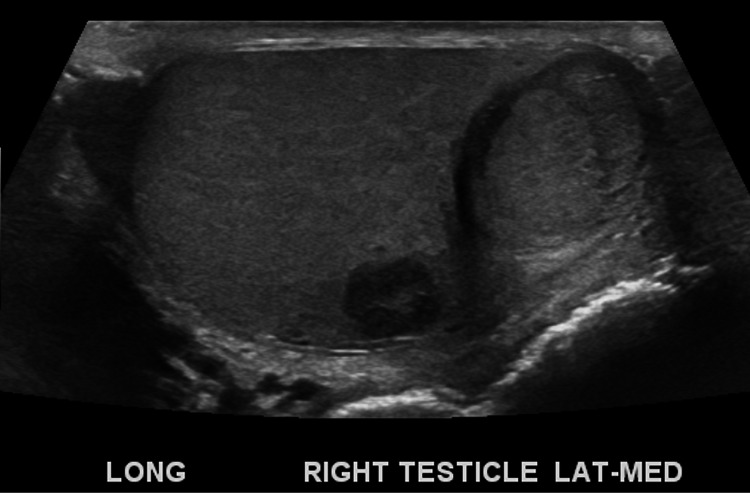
Right Testicular Masses on Ultrasound
